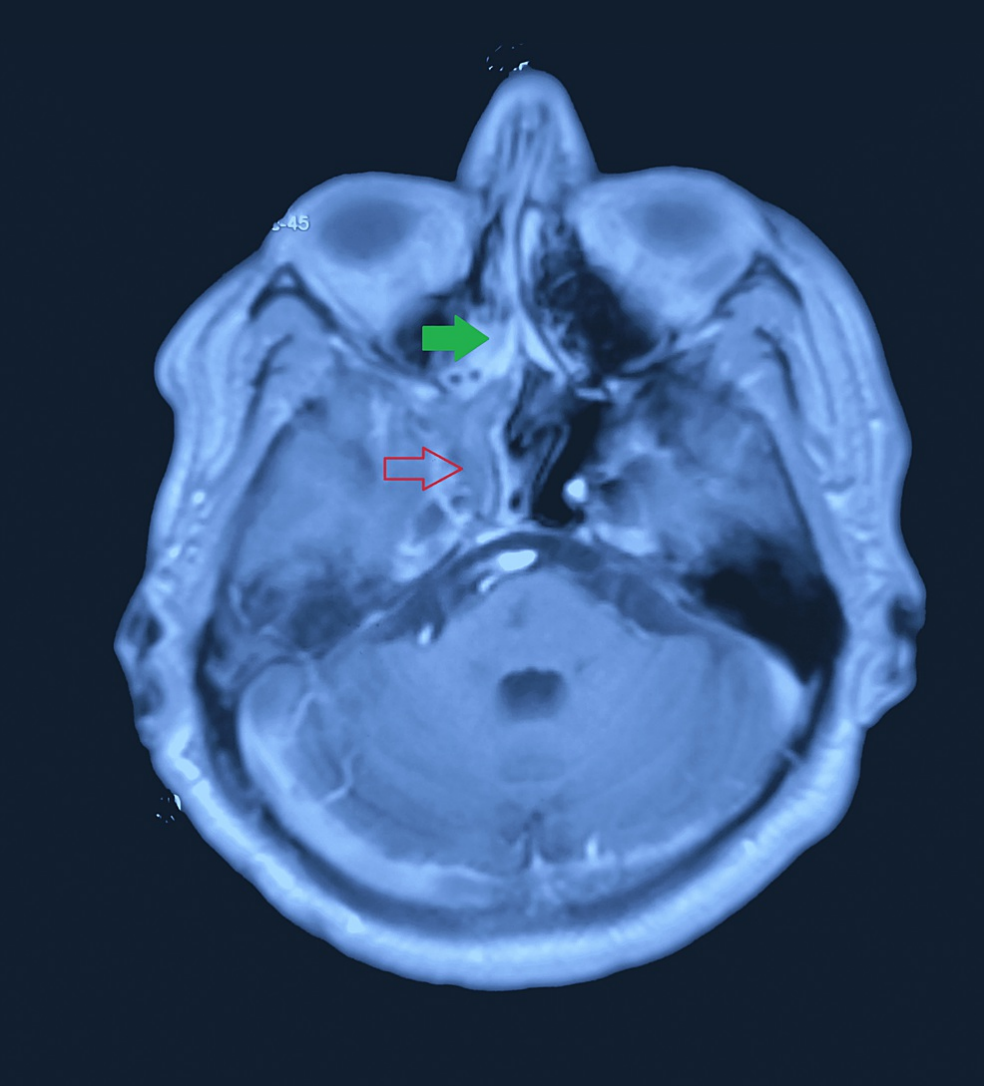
Contrast-enhanced MRI image showing mixed intensity lesion, with slight contrast enhancement involving the right maxillary and ethmoidal sinus (green arrow) extending into the right cavernous sinus region (red arrow). MRI: magnetic resonance imaging.
